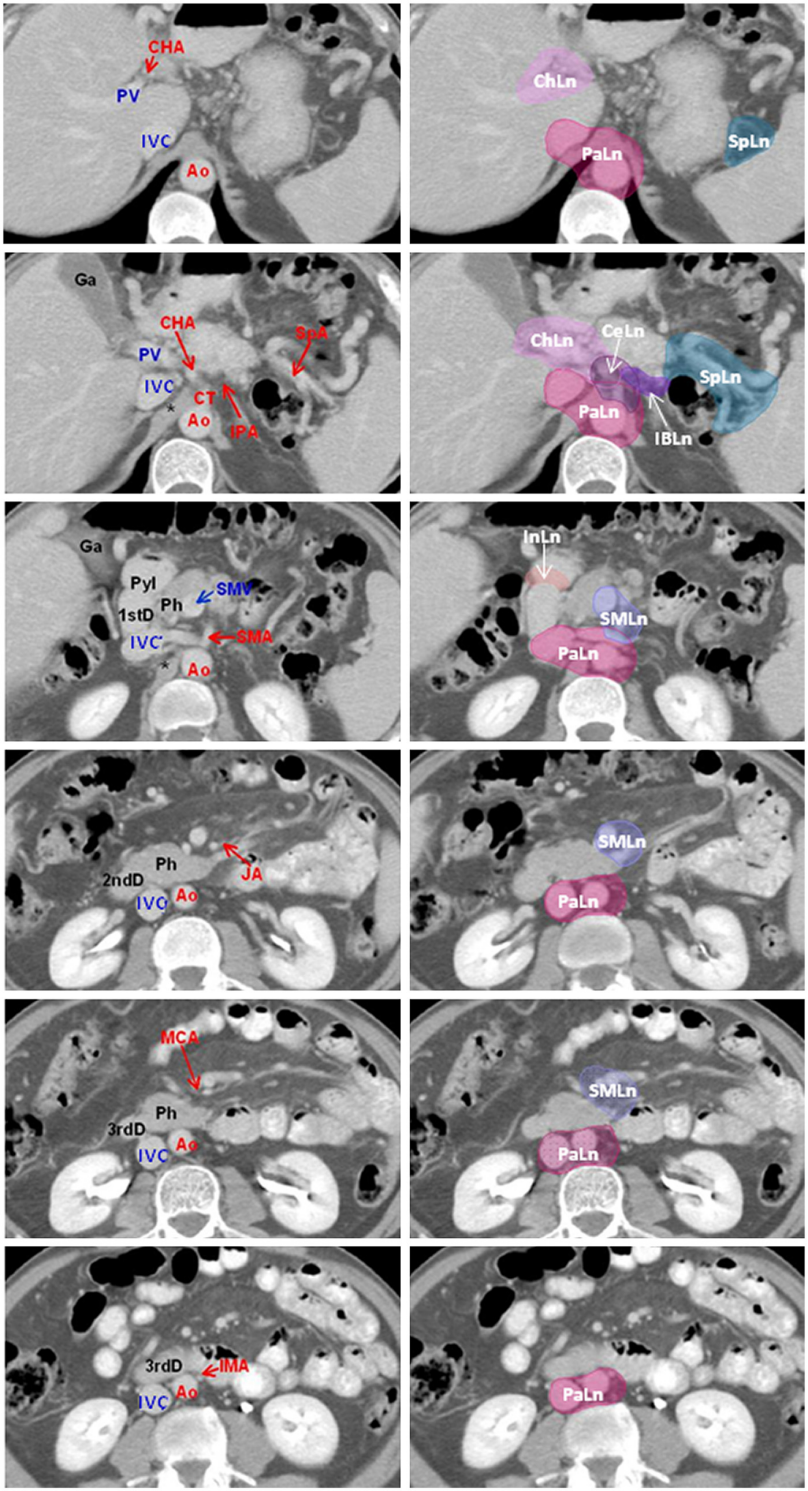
Anatomical structures for the high risk lymph nodal regions of the body/tail pancreatic cancer. Transverse CT slices through high risk lymph node regions of the body/tail pancreatic cancer from cranial to caudal direction. The abdominal artery and the anatomical structures were identified as a surrogate target for lymph node regions. A margin of 10 mm was added to the artery to delineate lymph node area, including the soft tissue with lymphatic and neural plexus. *Abbreviations*: Ao = Aorta; CeLn = Celiac Lymph nodes; CHA = Common Hepatic Artery; ChLn = Common hepatic and hepatoduodenal ligament Lymph nodes; CT = Celiac Trunk; 1st D = first part of Duodenum; Ga = Gallbladder; IBLn = Inferior Body Lymph nodes; IMA = Inferior Mesenteric Artery; InLn = Infrapyloric Lymph nodes; IPA = Inferior Pancreatic Artery; IVC = Inferior Vena Cava; JA = Jejunal Artery; MCA = Medial Colic Artery; PaLn = Paraaortic Lymph nodes; Ph = Pancreatic head; Pyl = Pylorus; PV = Portal Vein; 2nd D = second part of Duodenum; SpA = Splenic Artery; SpLn = hilus of the spleen and Splenic Lymph nodes; SMA = Superior Mesenteric Artery; SMLn = Superior Mesenteric Lymph nodes; SMV = Superior Mesenteric Vein; 3rd D = third part of Duodenum; * = pillar of the diaphragm.
